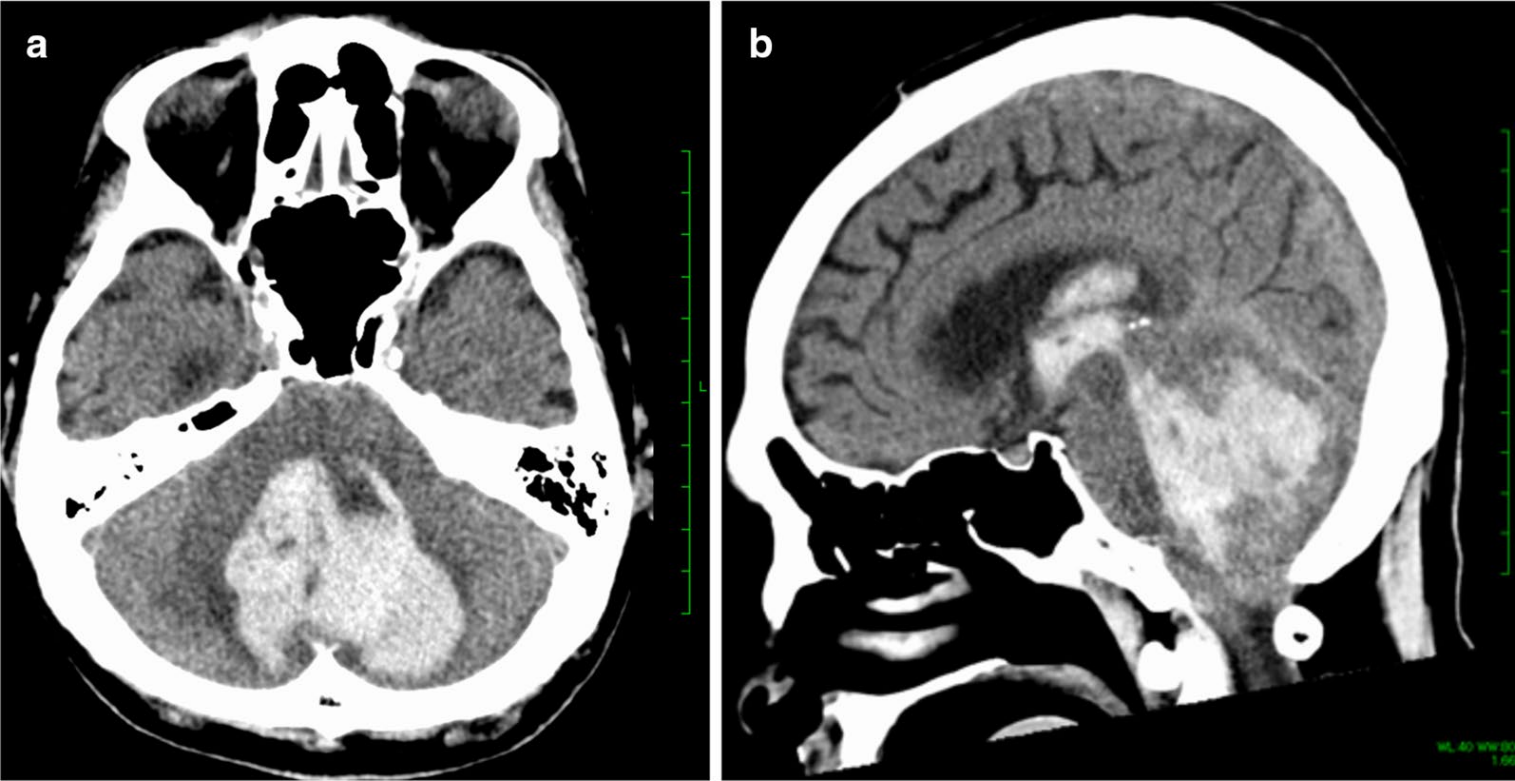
Brain computed tomography (CT) images of case 2. Cerebellar bleeding with intraventricular rupture was observed on POD 17
